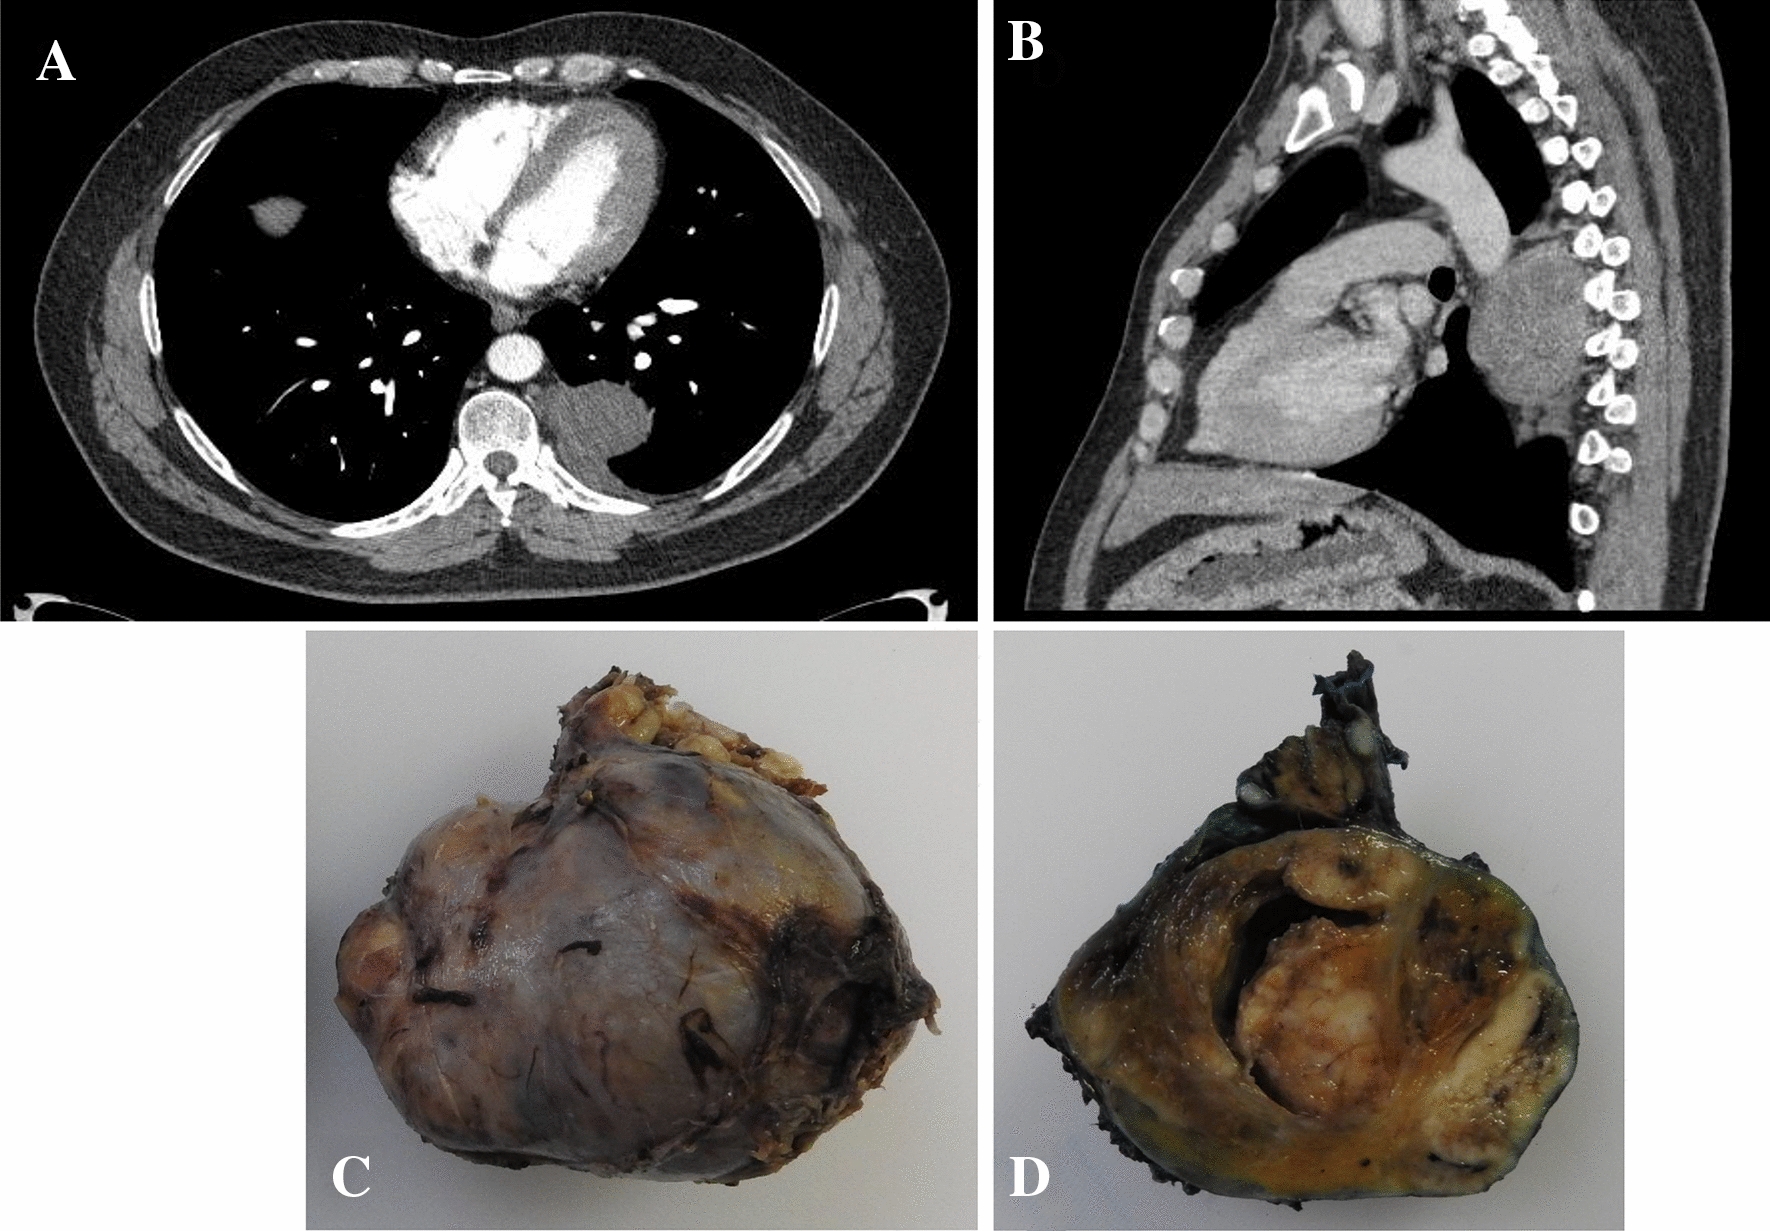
Benign mediastinal schwannoma. a, b CT images. The tumor was located in the posterior mediastinum and showed heterogeneous reinforcement with hypo and hyperintense areas after the application of contrast material; c Macroscopic images of the well-encapsulated ovoid tumor with a smooth surface, gray-yellow color; d Cross section of the tumor shows solid areas with cystic degeneration
